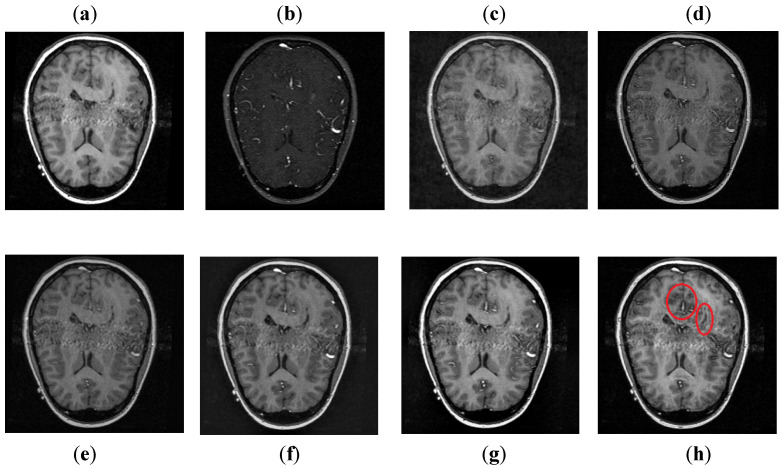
Data-2: (a) CT image, (b) MRI image, (c) DCT, (d) DWT, (e) NSST-SFT-PCNN, (f) CSR, (g) CNN, and (h) Proposed.
